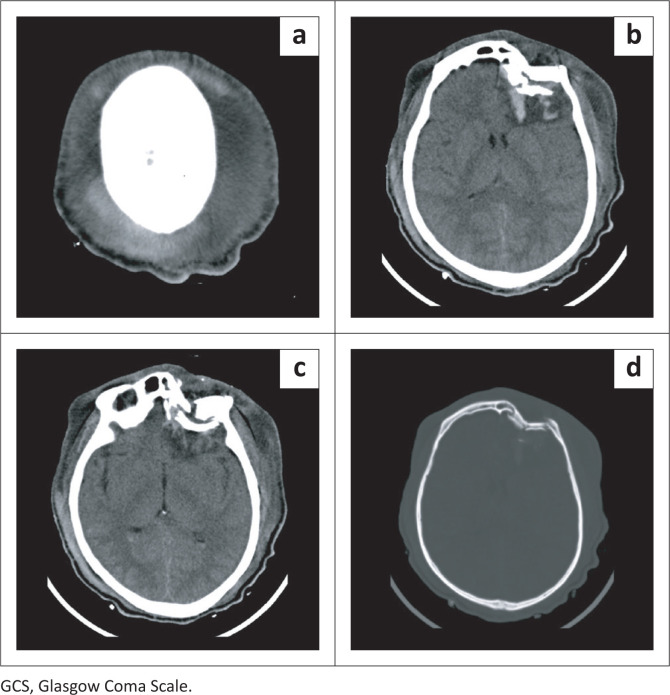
Twenty six-year-old male post fall from a moving vehicle with an initial Glasgow Coma Scale score of 7 received an axial unenhanced CT brain scan on brain (a, b, c) and bone window setting (d). CT imaging indicates extensive subgaleal soft tissue swelling (a), depressed comminuted left frontal skull fracture (b, c, d) with underlying intraparenchymal haemorrhages and perilesional oedema (b, c), left frontal subarachnoid haemorrhage (b, c) and intraventricular haemorrhage in the occipital horns of the lateral ventricles bilaterally (c).
